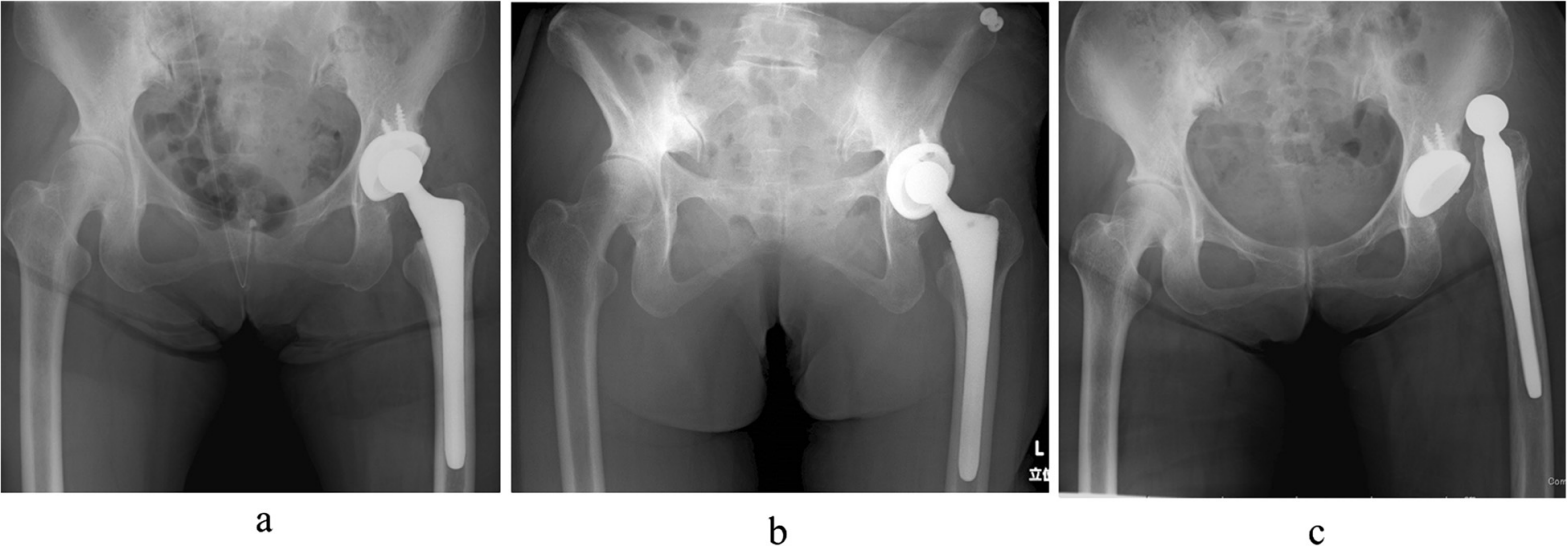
A 64-year-old woman with left hip osteoarthritis (after total hip arthroplasty). Anteroposterior pelvic radiographs taken after left total hip arthroplasty (THA) are shown. In the supine position (a), cup inclination is 45°, and cup anteversion is 25°. However, in the standing position, the pelvis markedly tilts backward; cup inclination is 59°, and cup anteversion is 42° (b). Although this case is not included in the present study, the case, in which the hip joint was anteriorly dislocated 2 weeks after THA (c), triggered the initiation of the study
